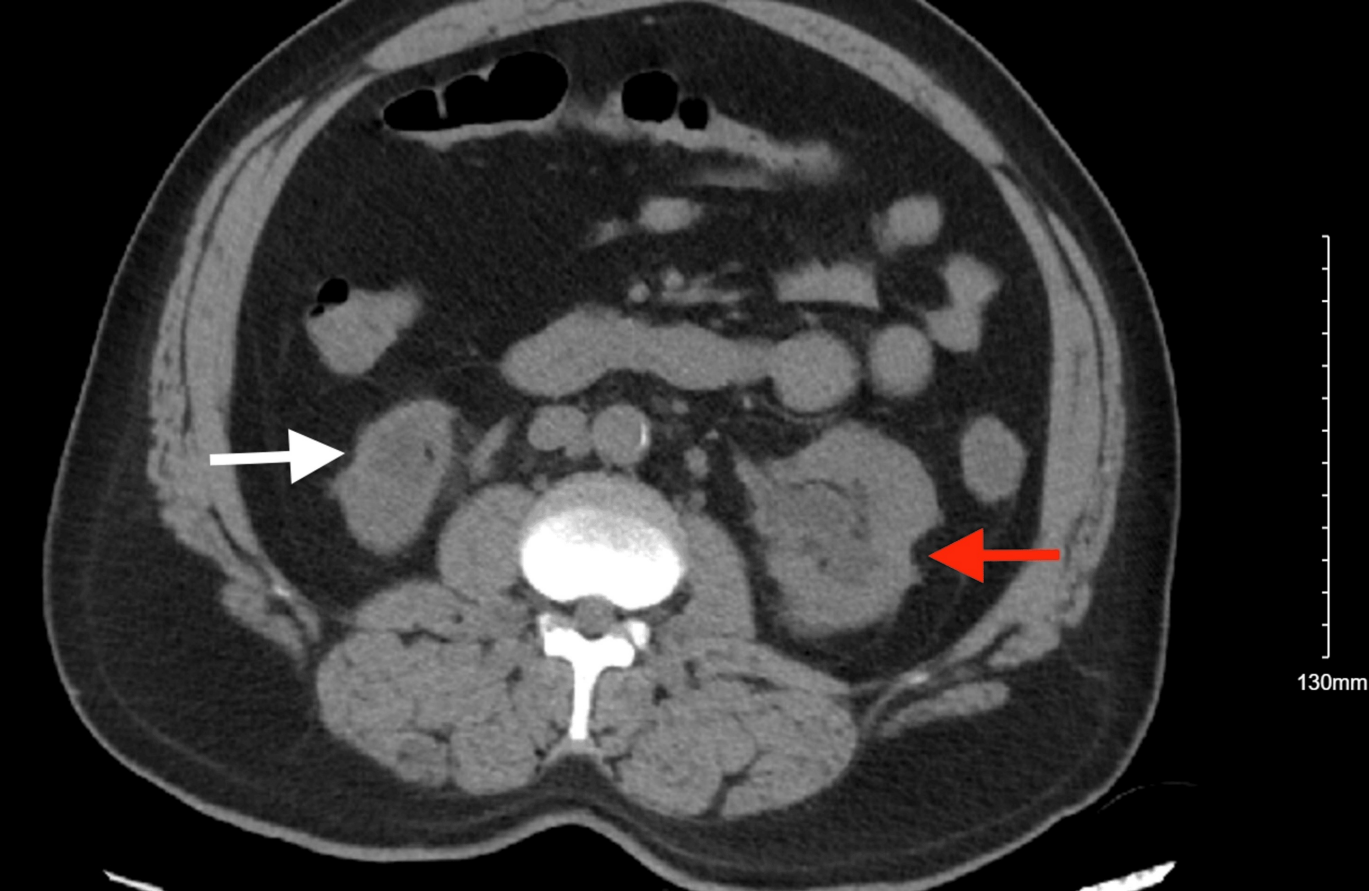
CT of the abdomen and pelvis (axial view) without contrast showing atrophic right kidney (white arrow) and left hydronephrosis (red arrow) with bilateral cortical scarring CT: computed tomography
